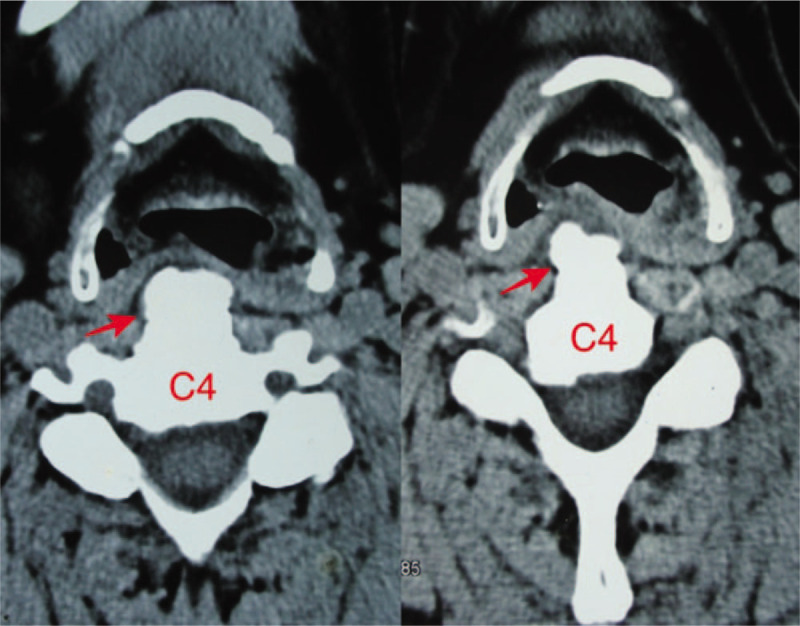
Axial CT slices through C4 in soft windows demonstrated the posterior wall of the pharynx was pushed to the pharyngeal cavity by large osteophytes (arrow). CT = computed tomography.
